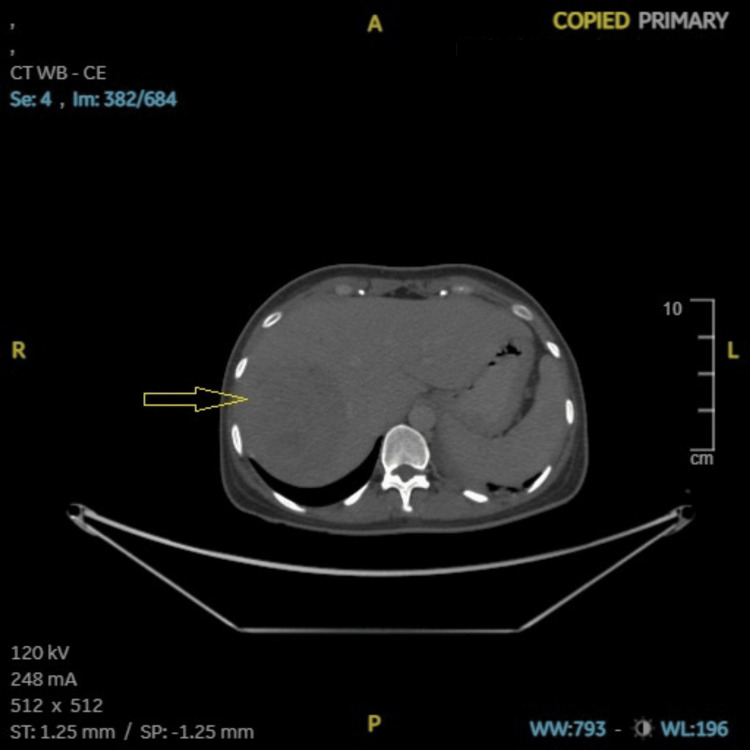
Case 2: CECT (abdomen and pelvis) showing hypoenhancing lesion in liver (arrow). CECT: contrast-enhanced computed tomography, A: anterior, R: right, L: left, P: posterior, ST: soft tissue, SP: soft tissue window (preset), WB: whole body, WW: window width, WL: window level.
